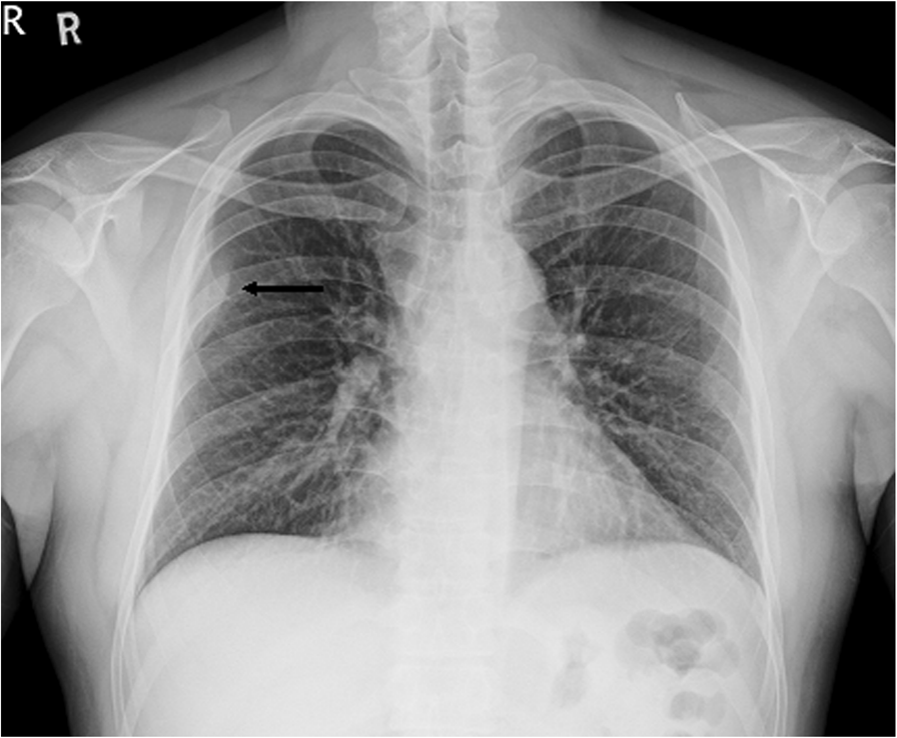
Chest X-ray of the patient. A chest X-ray showed a faint patchy opacity over the periphery of the right upper lung zone (black arrow).
